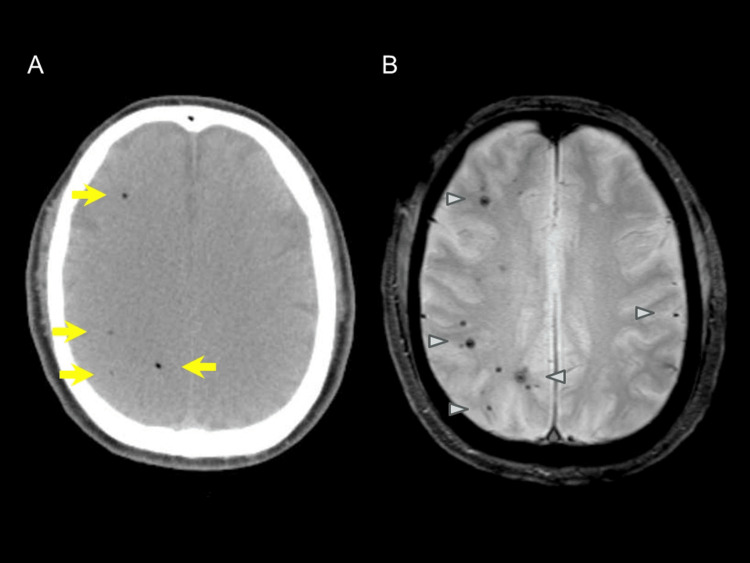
Brain CT and MRI on admission (Day 0). Brain CT on admission (Day 0) demonstrates multiple intravascular air foci within the right cerebral white matter (arrows), consistent with arterial cerebral air embolism (A). T2*-weighted MRI on Day 0 shows multiple hypointense spots (arrowheads) (B).
